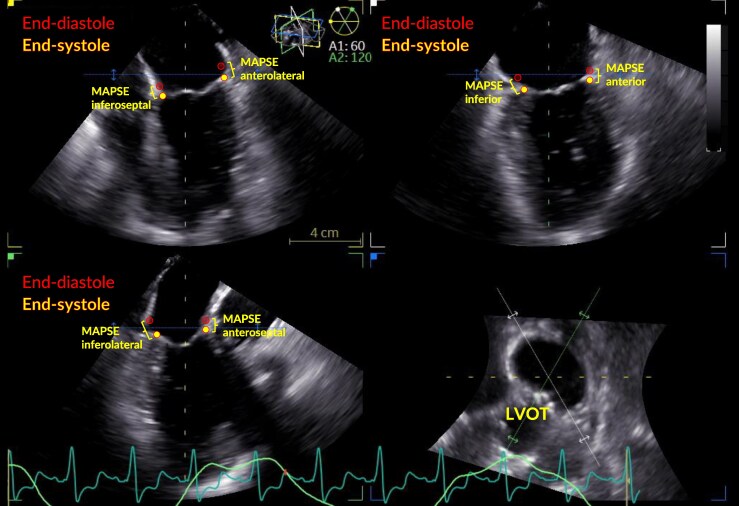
Manual measurement of MAPSE in a multiplanar reconstructed 3D transesophageal image (4D Views®, EchoPAC software version 204, GE Healthcare, Horten, Norway). *Filled points*: The mitral annulus at its lowest position at end-systole. *Open points*: The mitral annulus at its lowest position in a preceding frame. *Brackets*: MAPSE for each wall was measured as the absolute excursion. 3D manual MAPSE was calculated as the mean of all feasible measurements. LVOT, left ventricular outflow tract; MAPSE, mitral annular plane systolic excursion.
